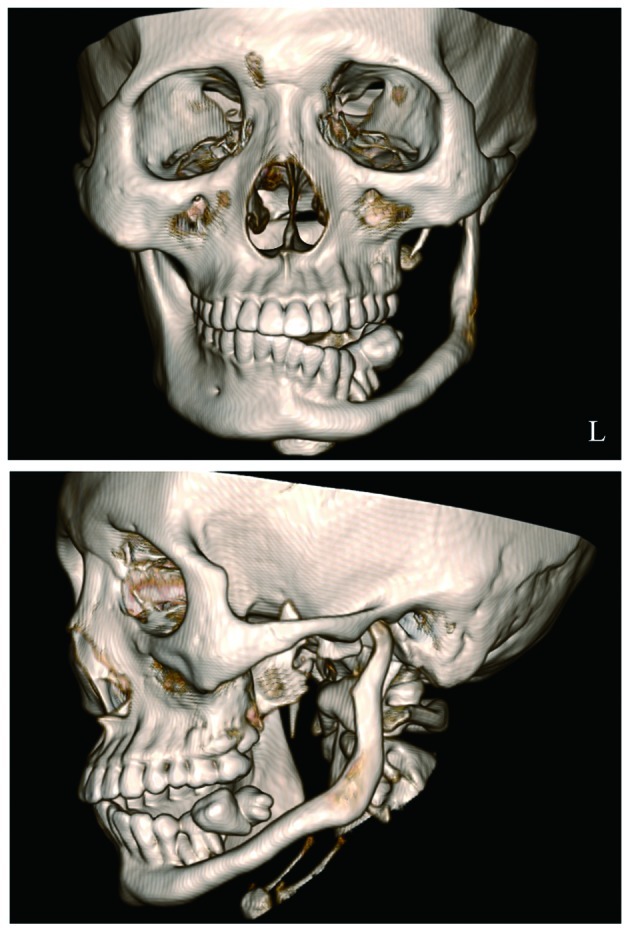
Three-dimensional reconstruction CT images of the maxillofacial region. Upper panel, front-view; Lower panel, lateral-view.
